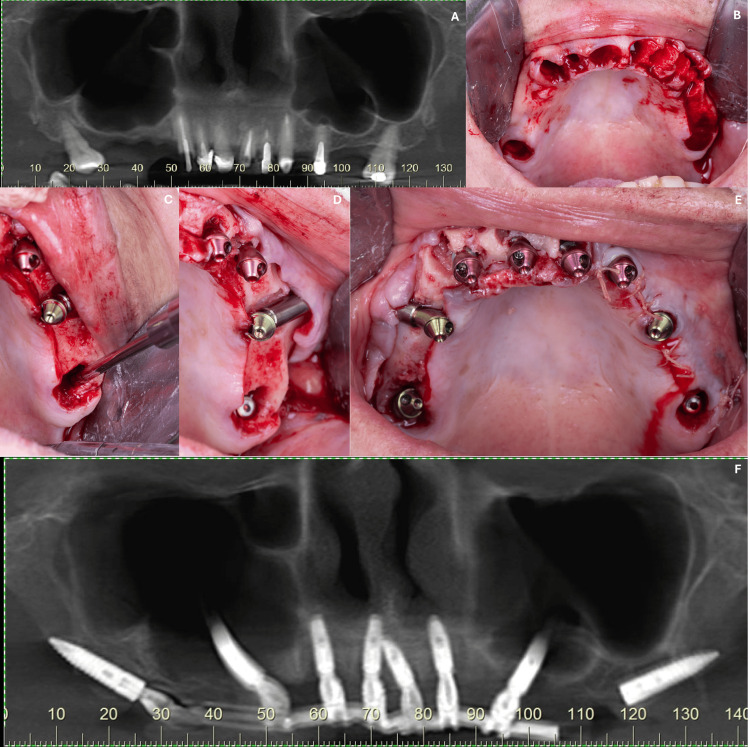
(A) Radiographic imaging showing teeth with inadequate direct and indirect restorations and large maxillary sinuses; (B) because of this, the total tooth extraction was indicated; (C-E) a full-arch maxillary rehabilitation supported by eight implants was proposed, including four conventional implants placed in the anterior region in fresh extraction sockets, two zygomatic implants, and two pterygoid implants; (F) radiographic condition of the prothesis supported by dental implants after 13 months of the implant loading
